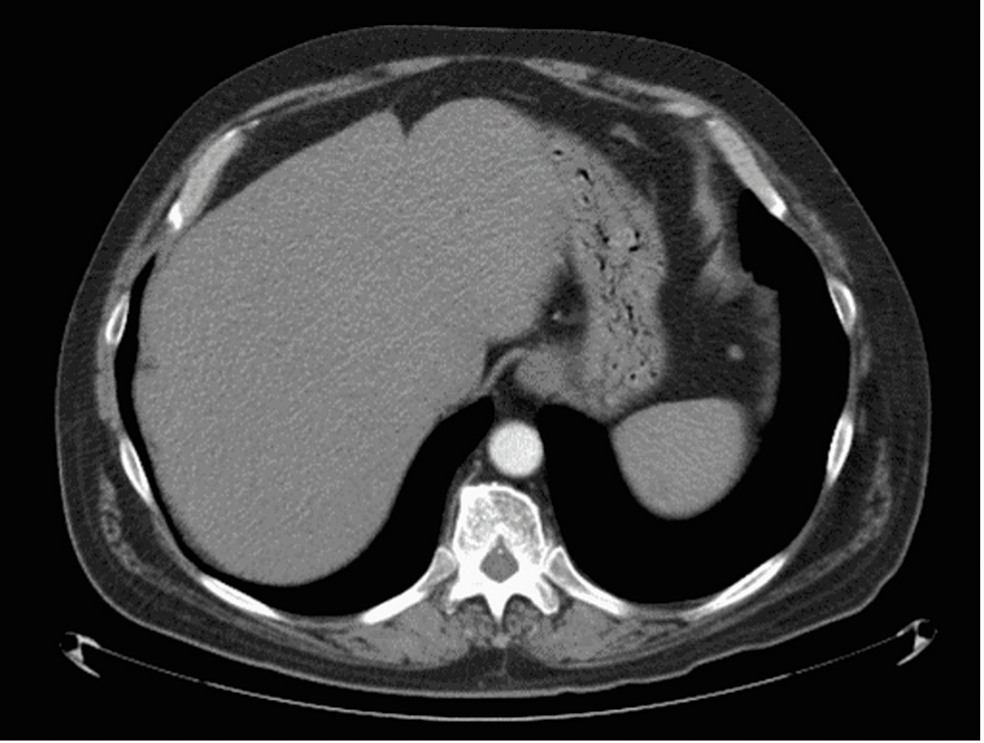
CT of the abdomen and pelvis in February 2022. CT of the abdomen and pelvis in February 2022 demonstrating resolution of previous liver lesions indicating stable disease. CT: computed tomography
